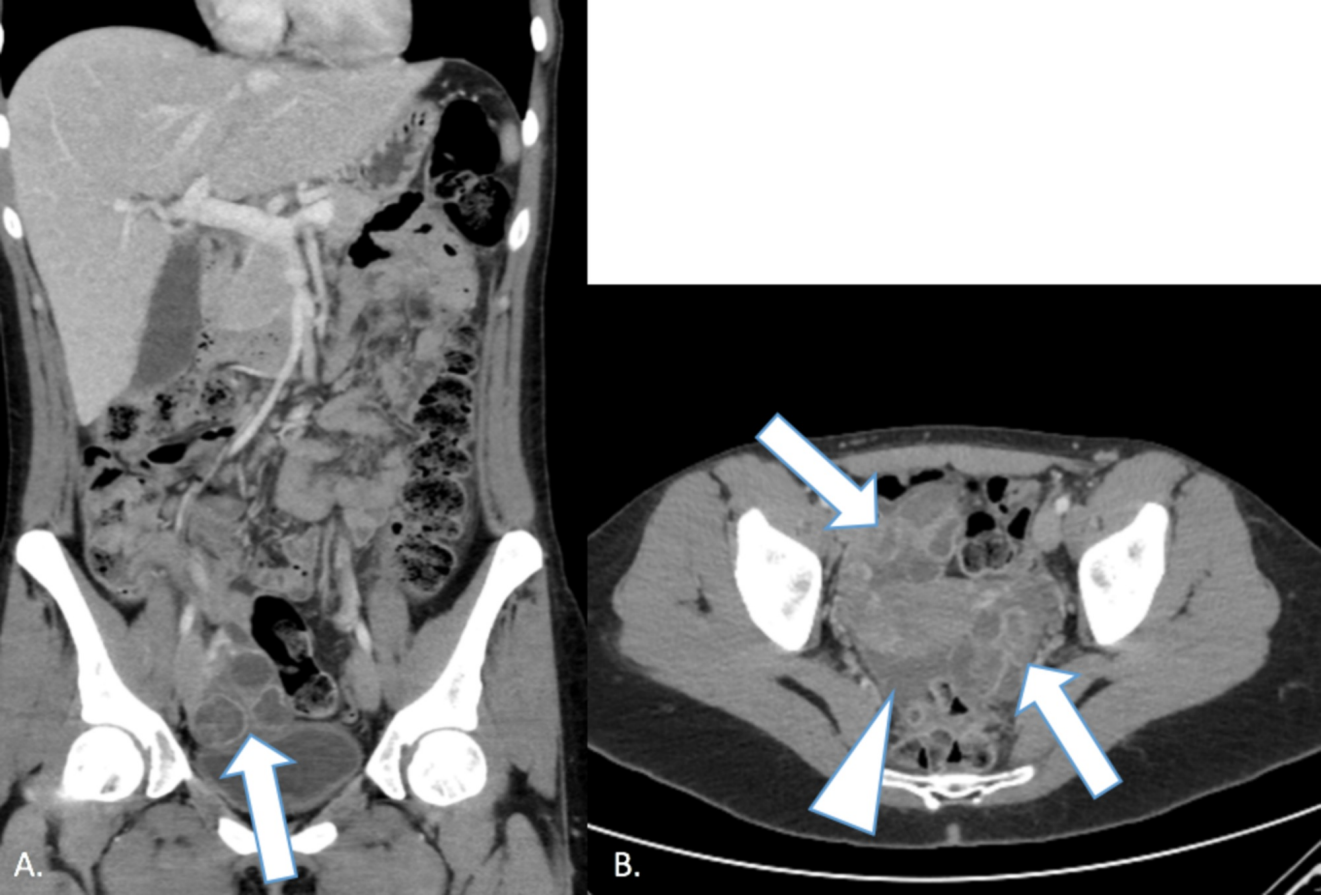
Computed tomography imaging of a 30-year-old female with a recent history of in vitro fertilization treatment showing findings consistent with mild grade 2 ovarian hyperstimulation syndrome. Image A is a coronal computed tomography image and image B is an axial computed tomography image that shows the patient's uterus with bilaterally enlarged cystic ovaries (arrows) and a small amount of fluid in the pelvis (arrowhead). These findings are consistent with the diagnosis of mild grade 2 ovarian hyperstimulation syndrome, as diagnosed on prior ultrasound imaging, with no other concurrent abdominal pathologies.
